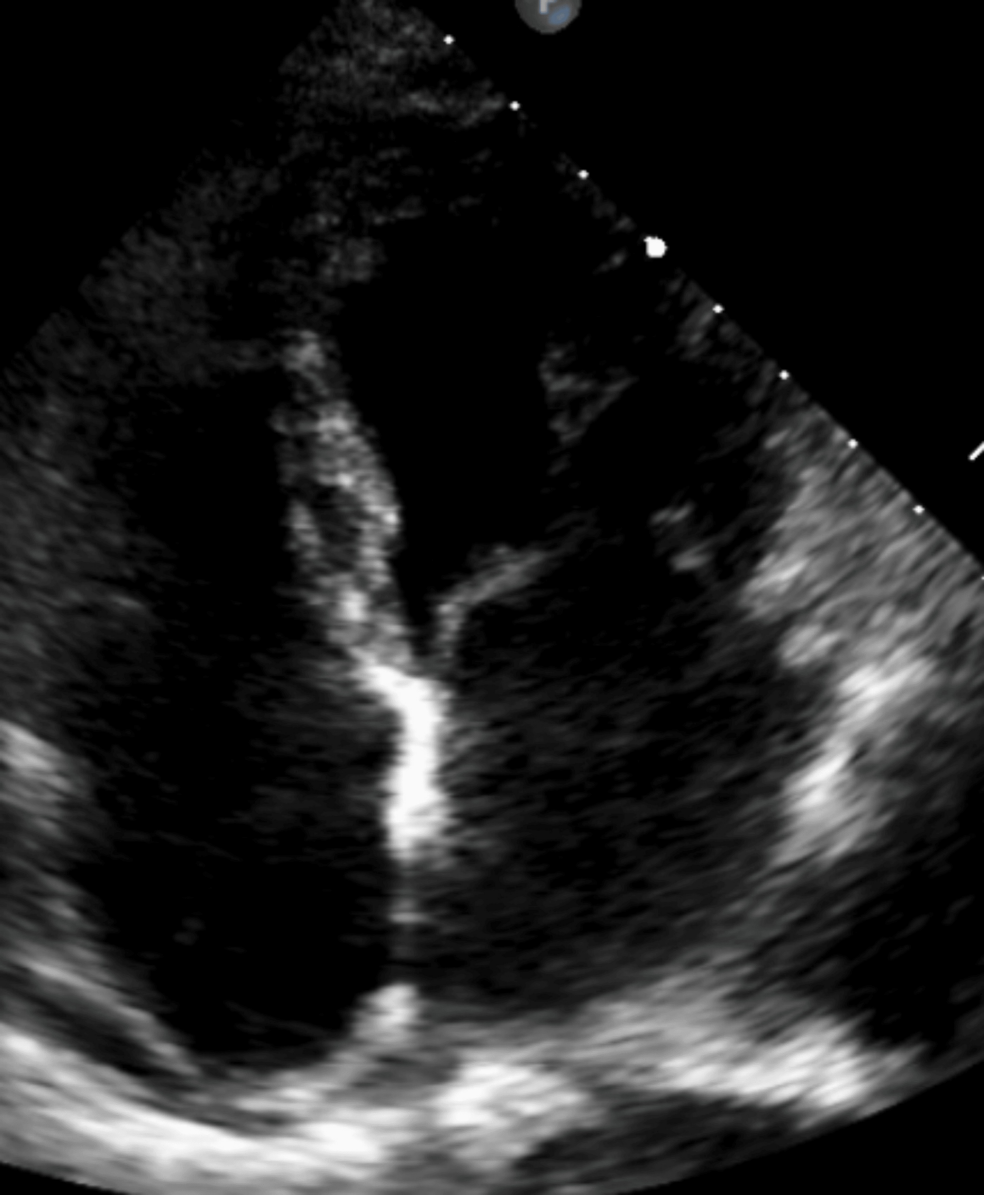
Transthoracic echocardiogram indicated mild pericardial fluid effusion
